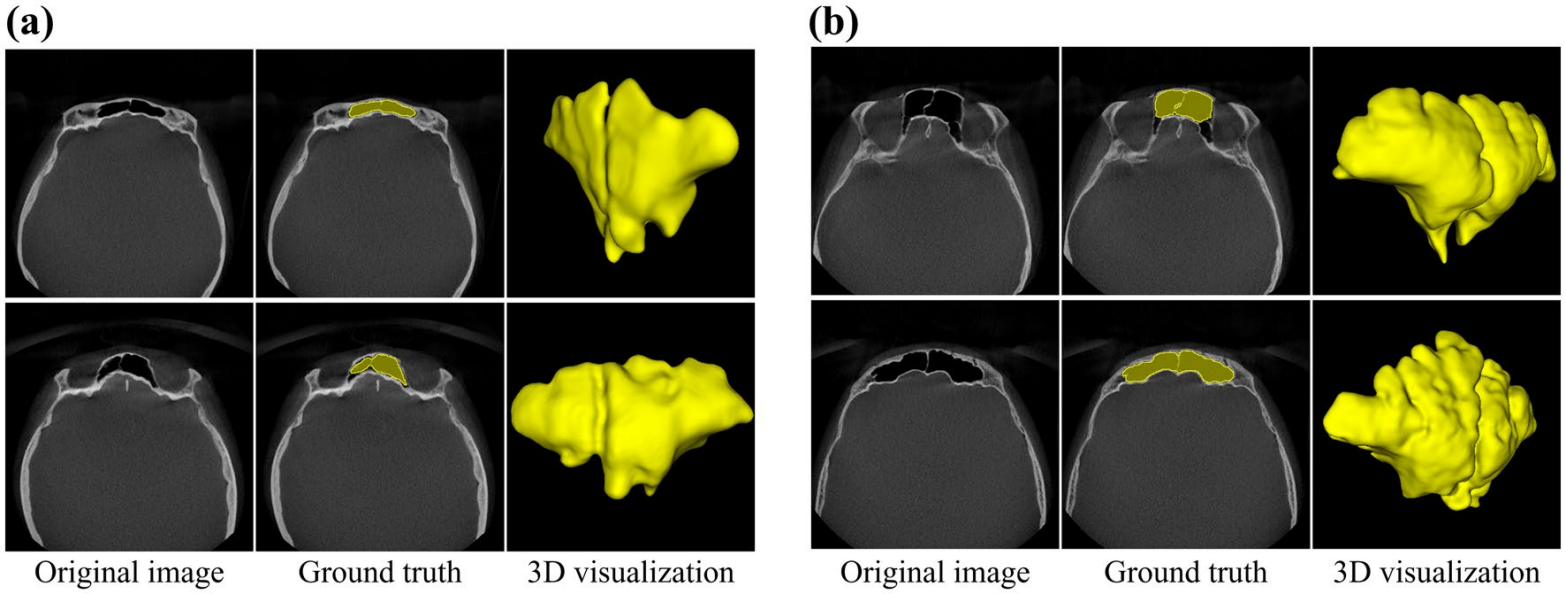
(a, b) CBCT images with label masks of the frontal sinus acquired from a female and male, respectively.
